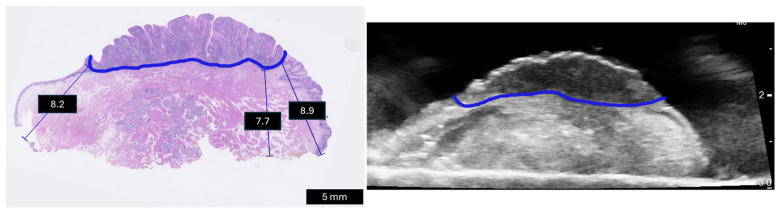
Pathology slice and its corresponding ultrasound frame. Tumor area is delineated by a pathologist and surgeon in blue on pathology and ultrasound, respectively. The pathologist measured the margins in mm.
